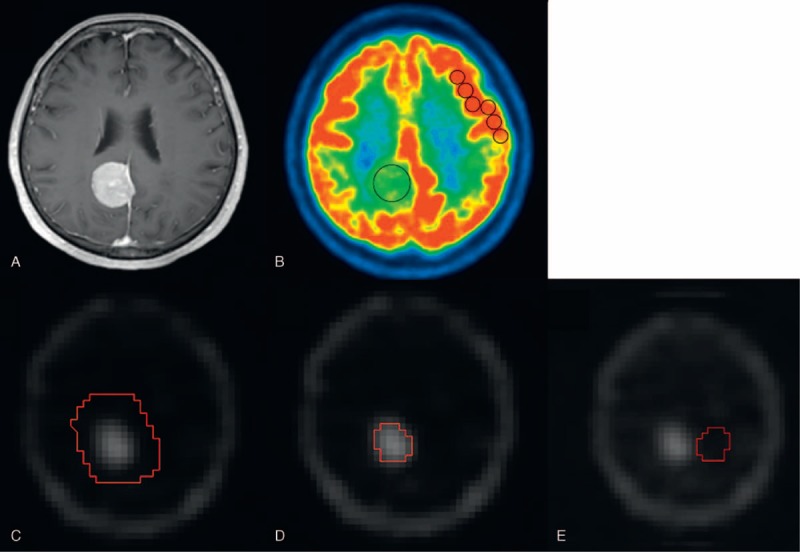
A representative analysis of a meningothelial meningioma (grade I), 70-year-old patient. Contrast-enhanced MRI (A) shows an enhanced extra-axial tumor at the right parietal region. FDG-PET (B) and Tl-SPECT (C–E) images show high uptake in the corresponding area. (B) On the FDG-PET image, ROIs were drawn on the tumor and contralateral gray matter. (C) On the Tl-SPECT image, the area corresponding to the tumor was encircled including the normal-appearing surroundings, but high uptake areas of the skull were excluded. (D) The tumor area way applying a threshold algorithm (Otsu method). (E) The tumor ROI was flipped horizontally, which was placed as the reference ROI at the contralateral normal brain of the same slice. By using these analysis procedures, SUVmax, SUVRmax, TURmean, and TURmax were measured as 5.75, 0.54, 6.84, and 9.30, respectively. FDG = fluorine-18 fluorodeoxyglucose, PET = positron emission tomography, ROI = region of interest, SPECT = single photon emission computed tomography, SUV = standardized uptake value, SUVmax = the maximum value of SUV, SUVRmax = the maximum SUV ratio, Tl = thallium-201, TURmax = the maximum Tl uptake ratio, TURmean = the mean Tl uptake ratio.
